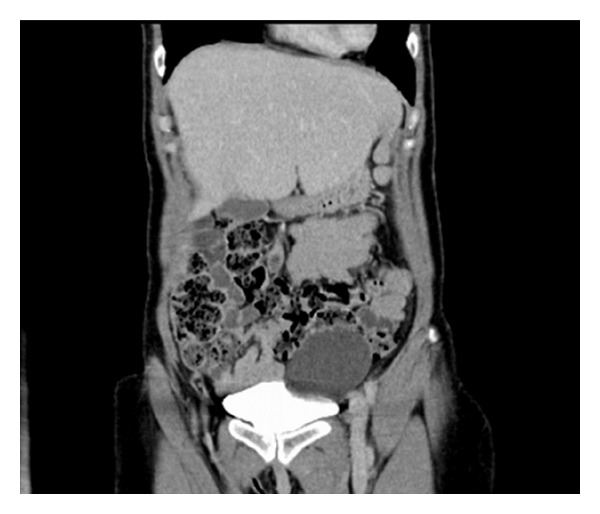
Coronal view of cyst showing its relationship with the sigmoid colon on computed tomography.
